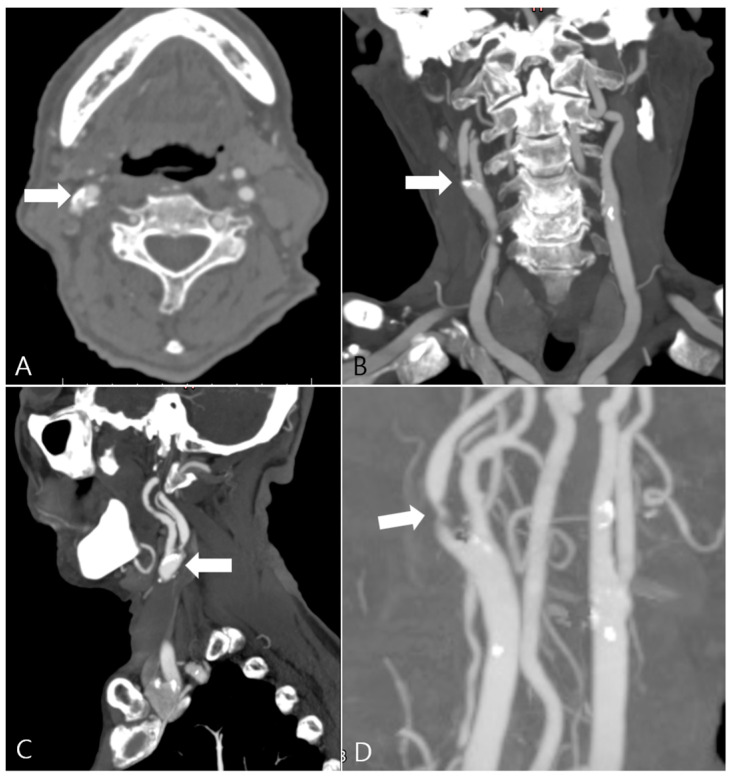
Representative case of carotid stenosis. Carotid computed tomography angiography (CTA) images of a representative patient with a carotid plaque causing severe stenosis in the right internal common carotid artery (arrows), visible in the axial plane (A), coronal plane (B), sagittal plane (C), and maximum intensity reconstruction (D).
